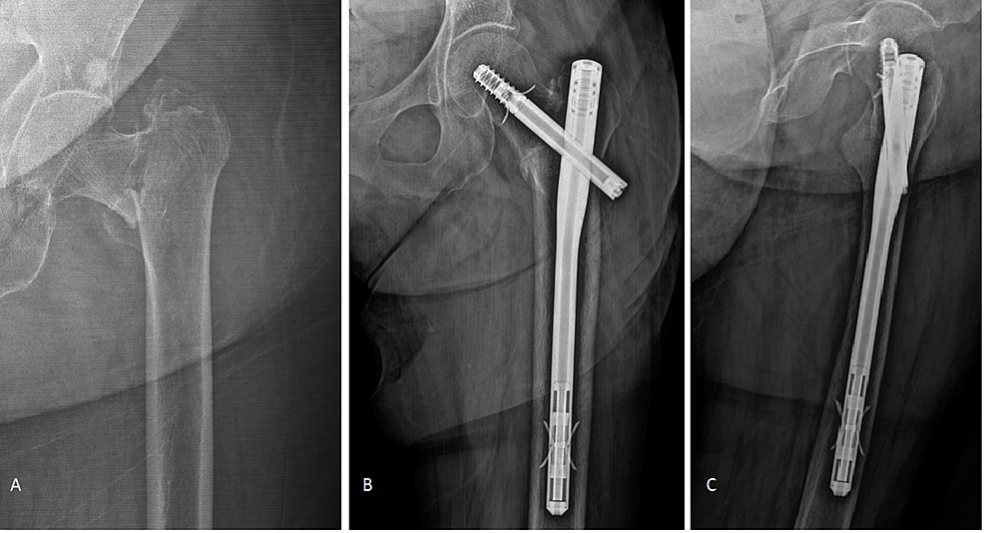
(A) Radiograph of a two-part basicervical fracture in an 86-year-old man. (B, C) Postoperative anteroposterior and lateral radiographs before completion of follow-up.
